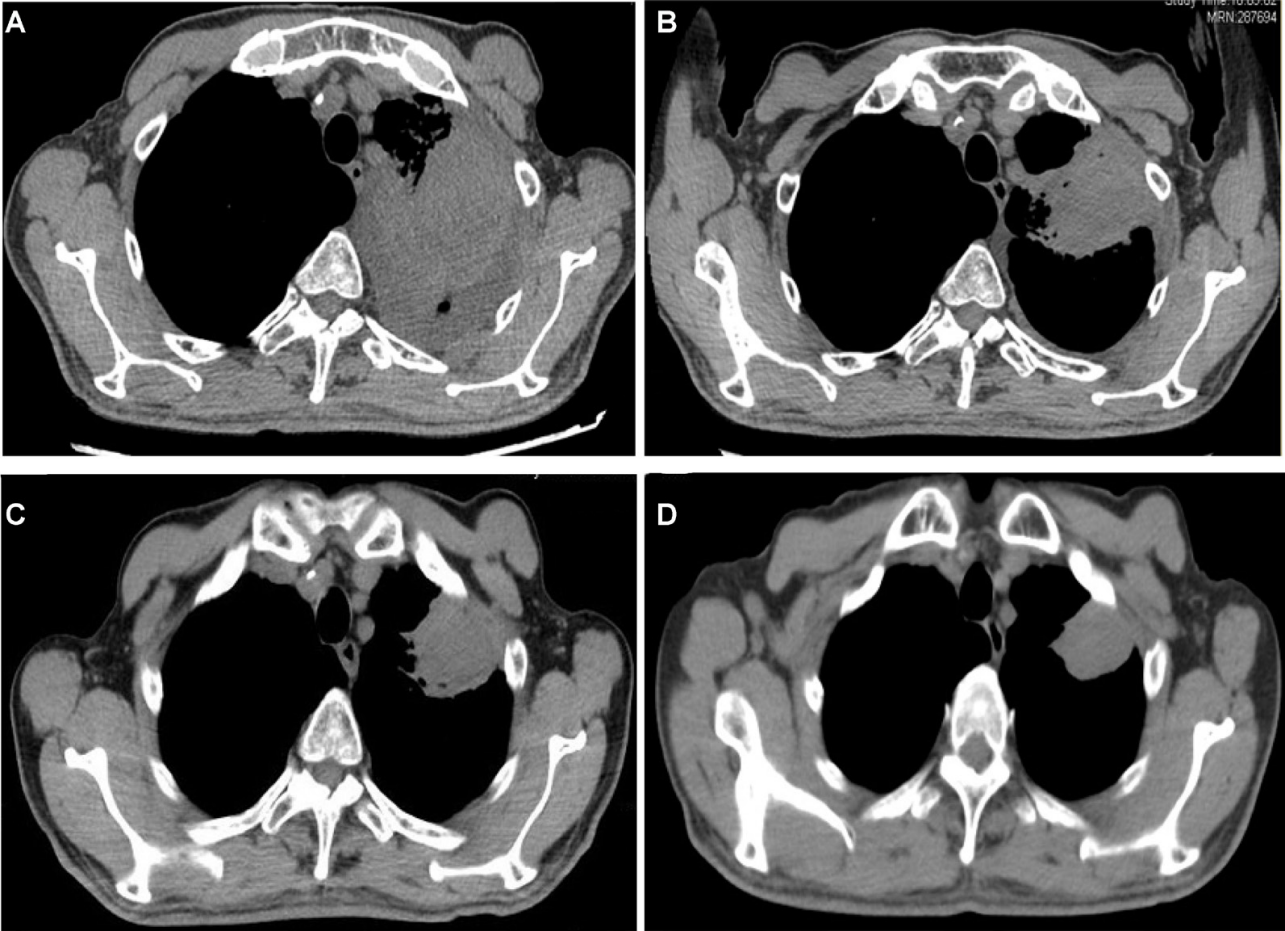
Chest CT showed tumor regression in the left lung after apatinib therapy (A–D) before and 1, 3, and 9 months after treatment, respectively.
